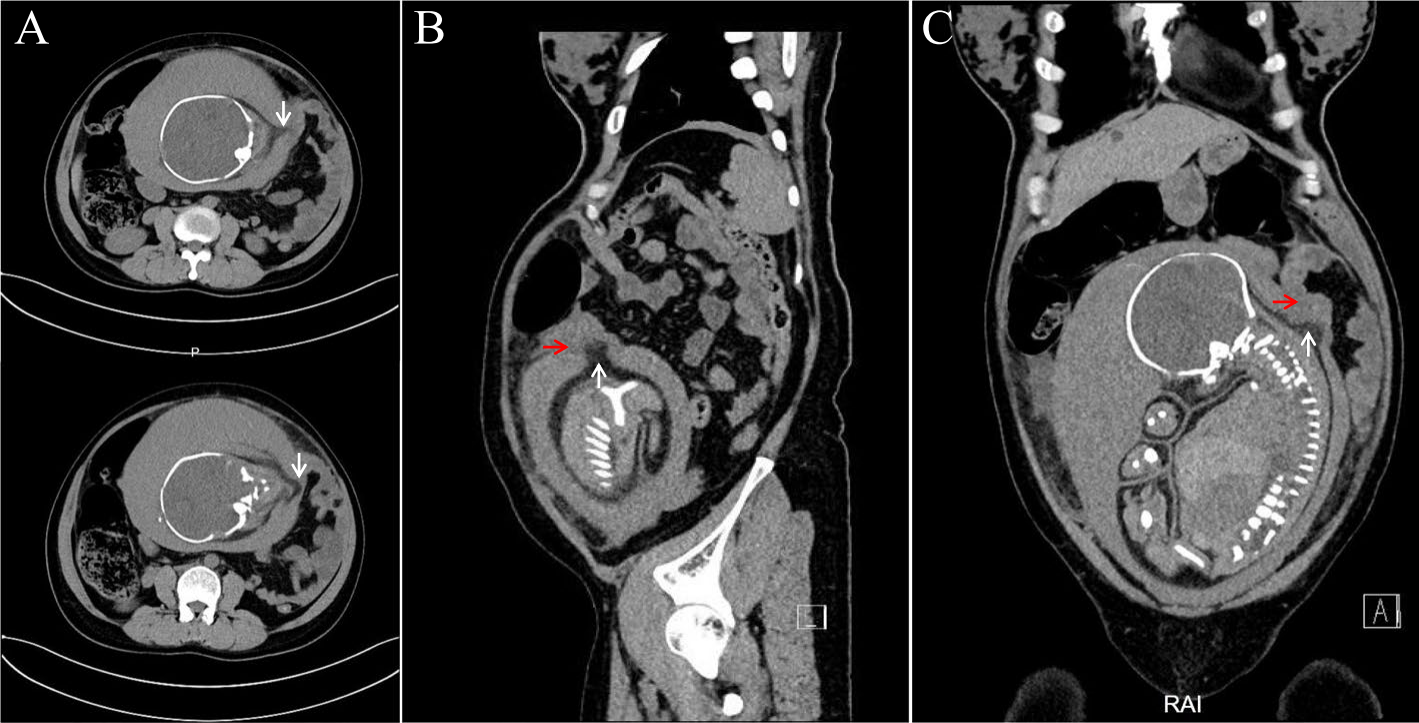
Axial (A), sagittal (B), and coronal (C) views of CT of the uterus. CT showing discontinuity of the uterine muscle layer on the left cornua (white arrow) and adhesion between the ruptured site and omentum (red arrow)
